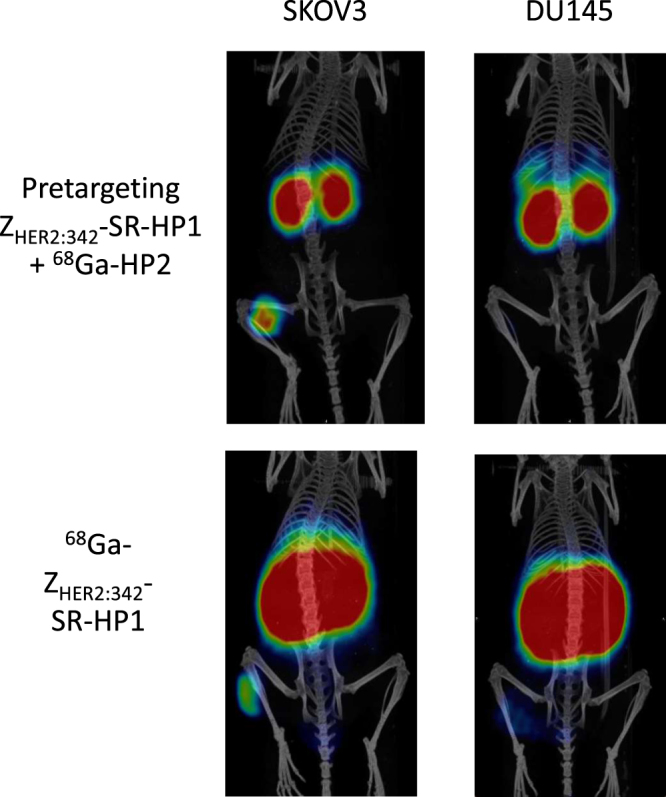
MicroPET/CT imaging of Her2-expressing SKOV3 (high Her2 expression) and DU145 (low Her2 expression) xenografts at 1 h after injection. Lower SUV threshold 0.07, upper SUV threshold 0.7.
